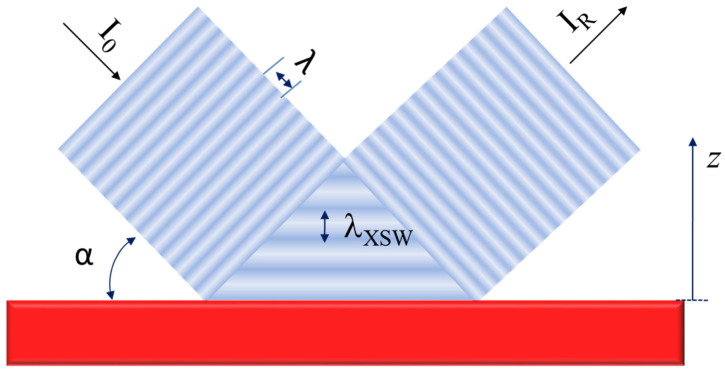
Cross-section of the X-ray standing waves field generated over a reflector’s surface below its critical angle. *I*_0_ and *I_R_* represent incident and reflected intensities. *λ* and λ_XSW_ describe the wavelength of the incident electromagnetic wave and the wavelength of the XRS field, respectively.
